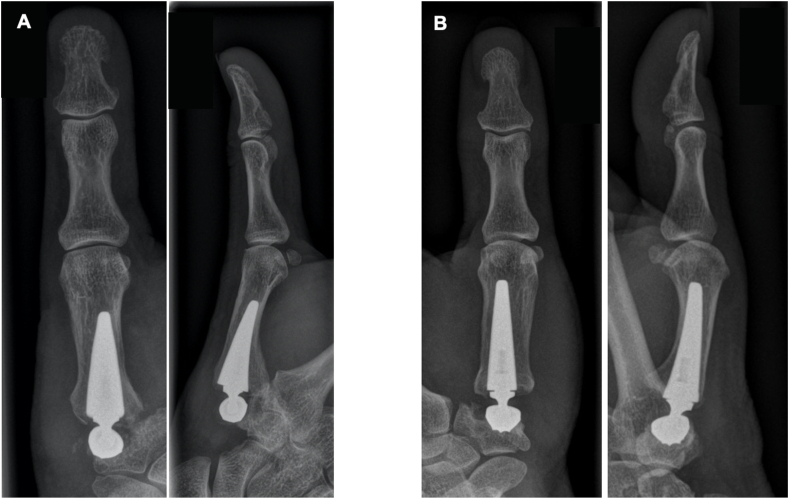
(A) Postoperative radiograph of a 56-year-old man presenting prosthesis dislocation with breakout of the trapezium. (B) Presentation of a bony cyst around the cup at 3-year follow-up after prosthesis implantation.
